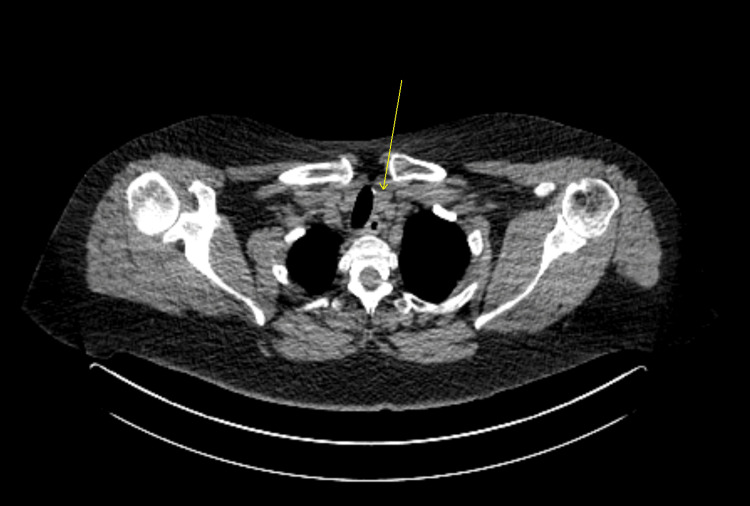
Increased metabolic activity in the left thyroid lobe on PET/CT scan, as indicated by a yellow arrow CT: computed tomography; PET: positron emission tomography
